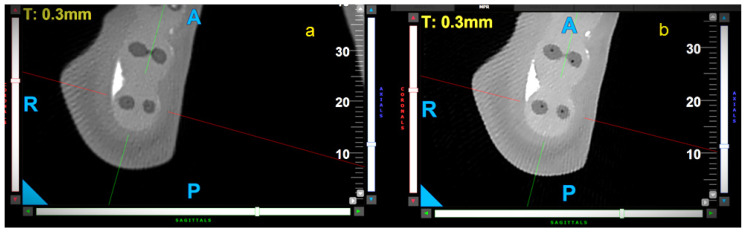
Representative CBCT axial—horizontal slices of samples at 3 mm from apex, before (a) and after (b) canal preparation—rotary movement. The letters A, P, R indicate sides—anterior, posterior, and right respectively.
